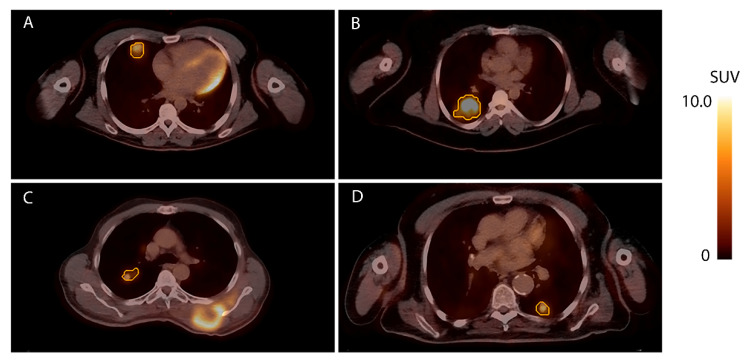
Examples of lung metastases with different metabolic characteristics. Axial planes of [^18^F]FDG-PET/CTs of four patients with lung metastases are shown with the corresponding VOIs outlined in orange. (A): A metastasis with a SUVmax of 6.1 detected 22 months after resection of an undifferentiated soft tissue sarcoma in the right deltoid muscle. (B): A metastasis with a SUVmax of 9.0 detected 34 months after resection of an undifferentiated soft tissue sarcoma in the right gluteus maximus. (C): A metastasis with a SUVmax of 5.2 detected synchronous with a myxofibrosarcoma originating from the left thoracic wall. (D): A metastasis with a SUVmax of 7.2 detected synchronous with local recurrence of a leiomyosarcoma in the right lower leg.
